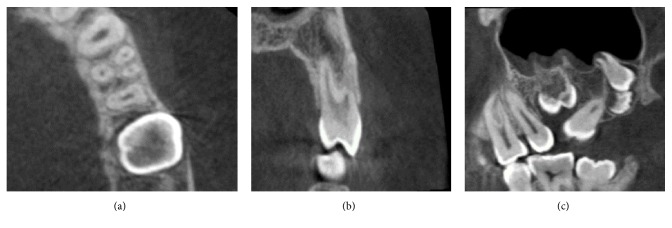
CBCT of maxillary first premolar with three roots: (a) the axial plane, (b) the coronal plane, and (c) the sagittal plane.
